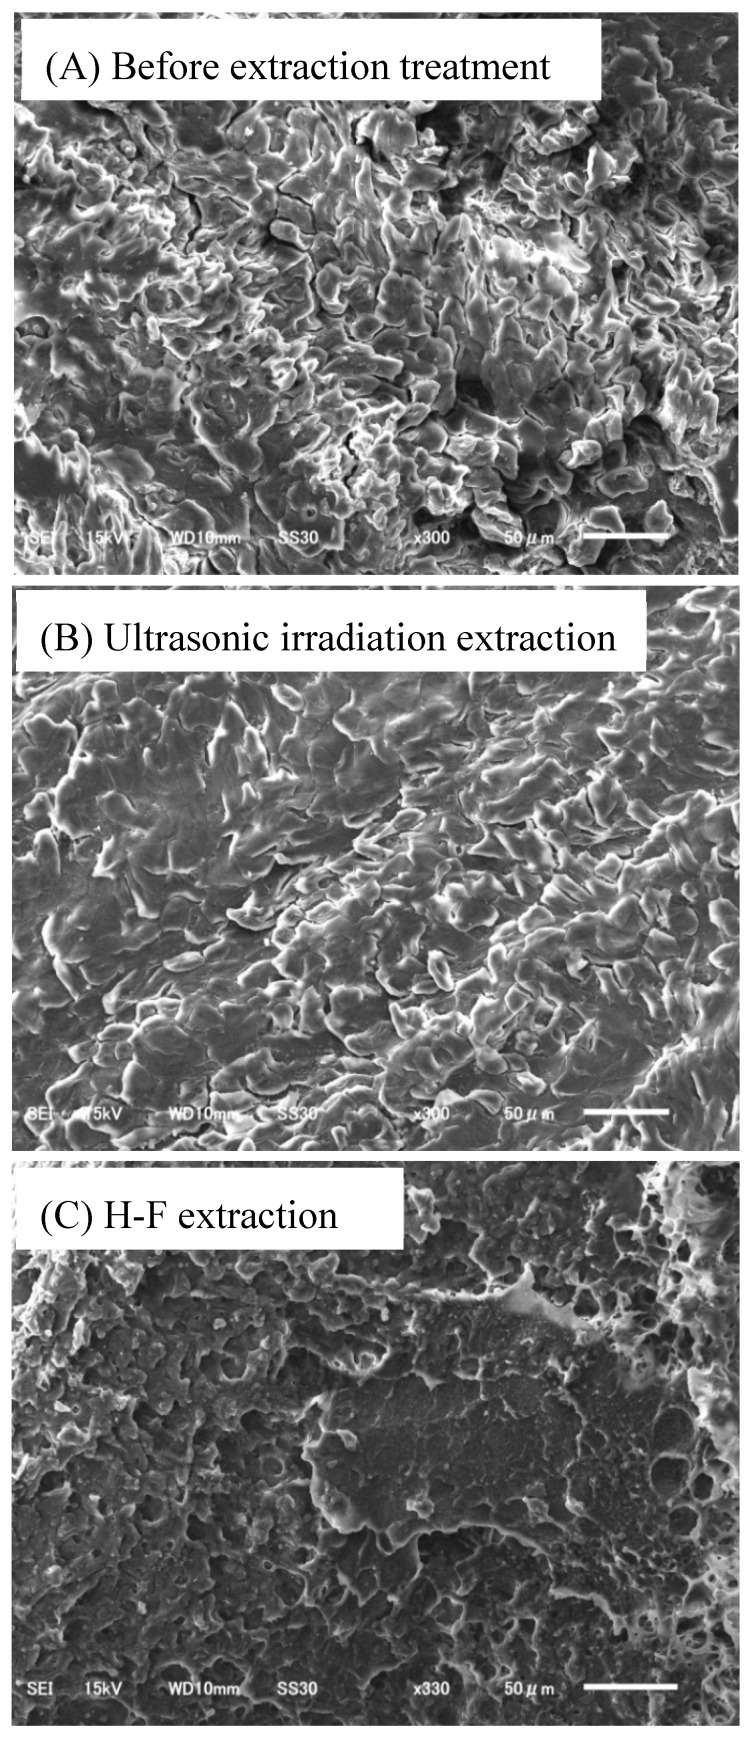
SEM images of the nail surface (A) before the extraction treatment, (B) after ultrasonic irradiation extraction (30 min), and (C) after H-F heating extraction (3 min).
